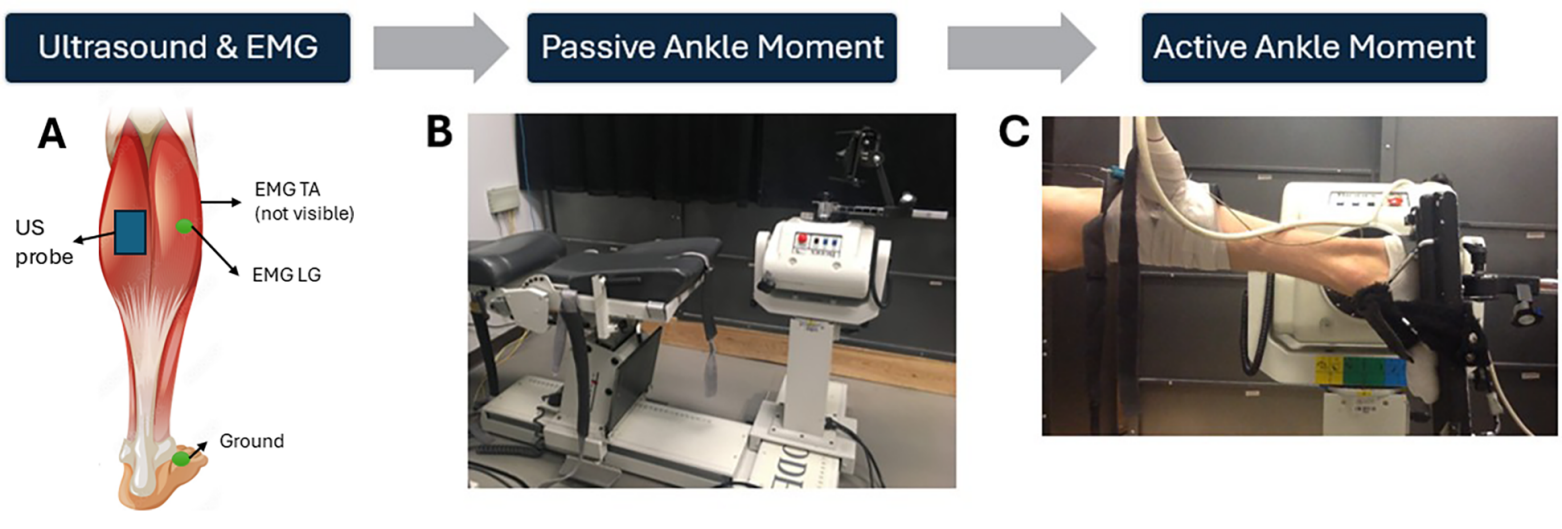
Experimental set-up. Placement of the ultrasound probe on the MG and sEMG electrodes on the LG and TA muscles, respectively (A) The measurement of passive moment, avoiding any passive moment due to the weight of the foot and gravity, by having participants side-lying during the passive assessments (B) Measurement of active ankle plantar flexor moment with the participant lying prone. (C) Adapted with permission from Muscle Stock Vector | Adobe Stock, File # 83745249 by blueringmedia, licensed under standard license, stock.adobe.com.
